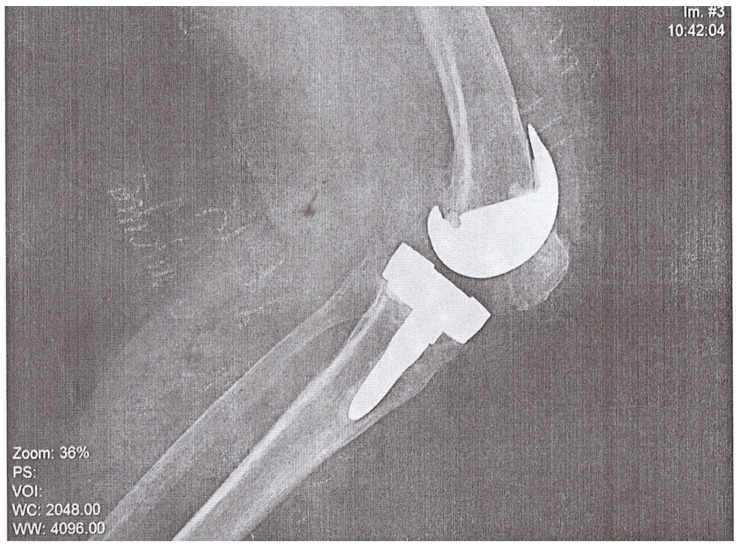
Lateral radiography, pre-operative, of suspected left knee PJI.
